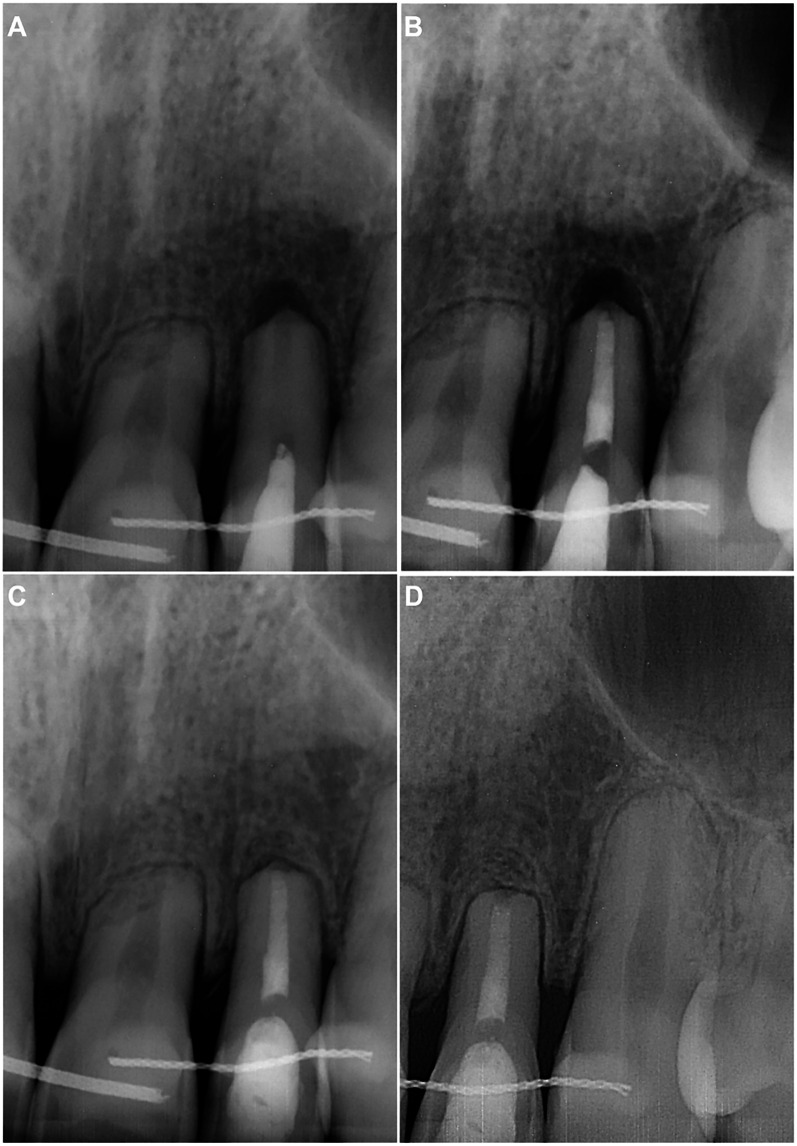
(A) Preoperative periapical radiograph demonstrating the empty root canal and periapical lesion of tooth 22. (B) Postoperative periapical radiograph after the root canal filling with ProRoot MTA White, followed by a wet cotton pellet and temporary restorative material IRM in the endodontic access cavity. (C) Six-month follow-up periapical radiograph showing a completely healed periapical lesion on tooth 22. (D) Eighteen-month follow-up periapical radiograph demonstrating no pathological changes in the tooth and periapical tissues.
